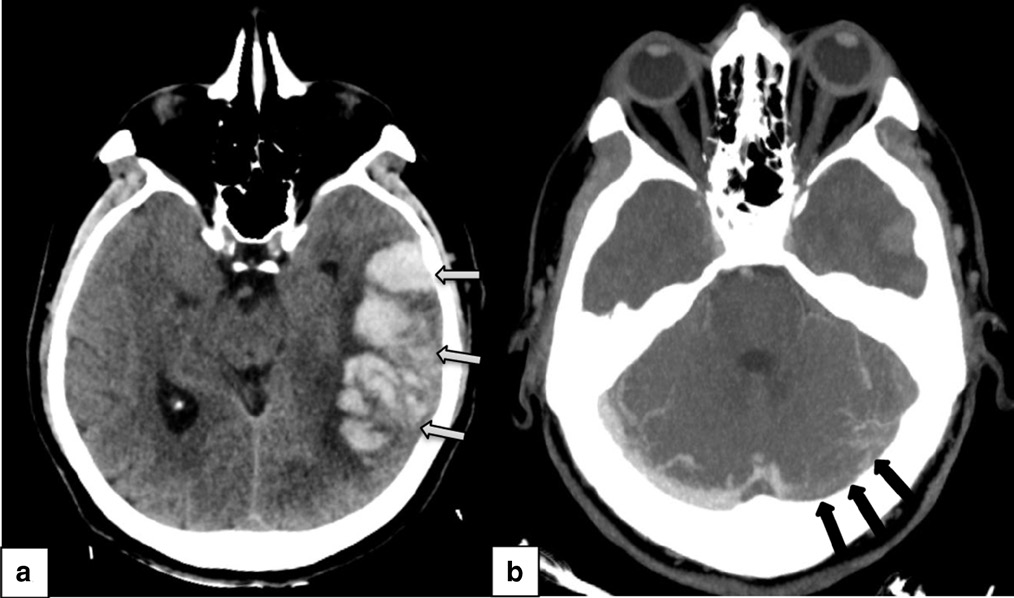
CT scan of the head (a) shows a large left frontotemporoparietal hemorrhage (grey arrows) with surrounding edema causing mass effect over the left lateral ventricle and surrounding sulci. CT venogram (B) shows thrombosis of the left transverse sinus (black arrows).
